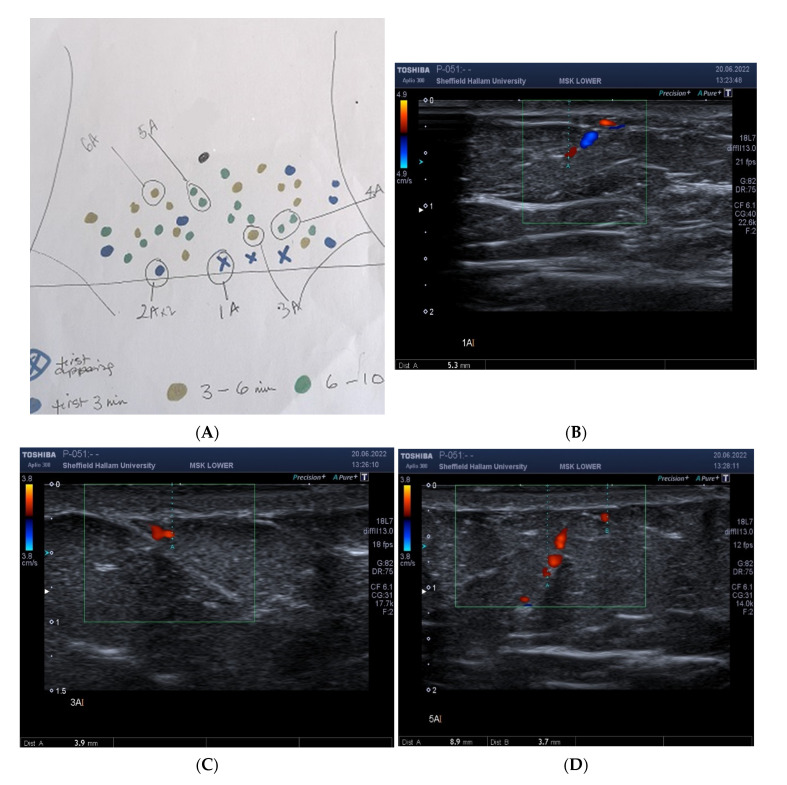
Correspondence of hot spot locations to guide position of Doppler ultrasound probe. Location of abdominal hotspots as they appeared in real-time, and mapped manually, as a sketch at the bedside, during 10 min video thermography (A). The labels were drawn to identify the sequence of appearance and to guide the sonographer for placement of the ultrasound probe. Blue marker (X) indicates first spot/s to appear. Blue circles, hot spot appearance within the first 3 min; gold, 3–6 min; green, 6–10 min, A. In this subject, three of the first hot spots appeared simultaneously and were marked accordingly by blue (X). Depth of vessel (5.3 mm) at hotspot (1A) measured using colour and power Doppler Ultrasound, (B). Vessel depth at 3 A 3.9 mm, (C). Depth of vessels at spots 5 A, 8.9 mm and 3.7 mm, respectively, (D).
